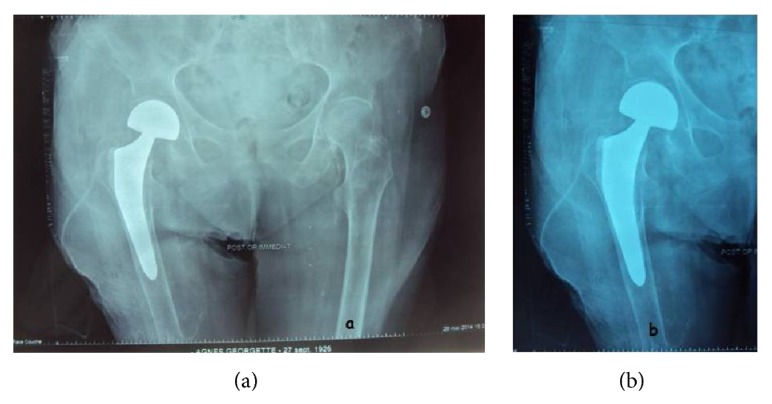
Postoperative radiography with uncemented bipolar hemiarthroplasty.
